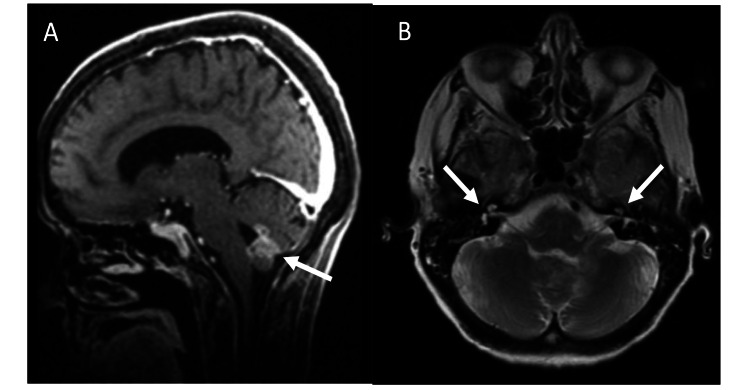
MRI brain with gadolinium (A) Sagittal image of T1 brain MRI with gadolinium with white arrows demonstrating heterogeneously enhancing lesion at the area postrema. (B)  Axial image of T2 brain MRI with gadolinium with white arrows demonstrating enhancement of cranial nerves VII and VIII.
